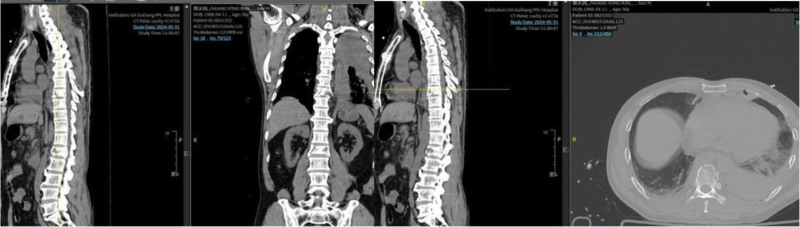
Computed tomography (CT) images of the thoracic and lumbar spine on admission. CT = computed tomography.
